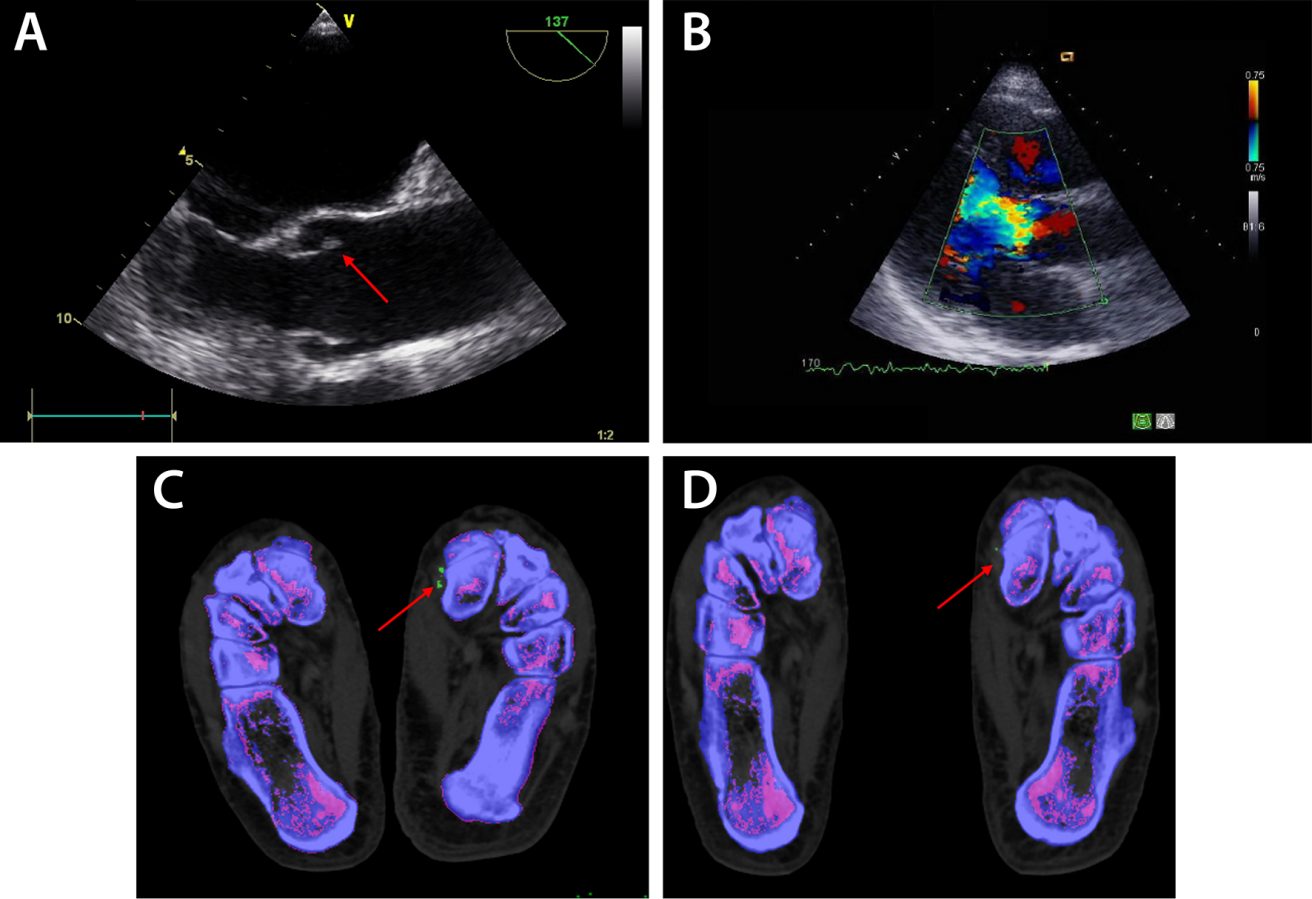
A, B Two-dimensional transesophageal echocardiography, suspected endocarditis on aortic valve (red arrow) with severe regurgitation seen on color flow imaging (B). C Urate deposition (green dots marked by red arrow) in dual-energy CT. D Five months later, urate depositions (red arrow) were partially reduced
